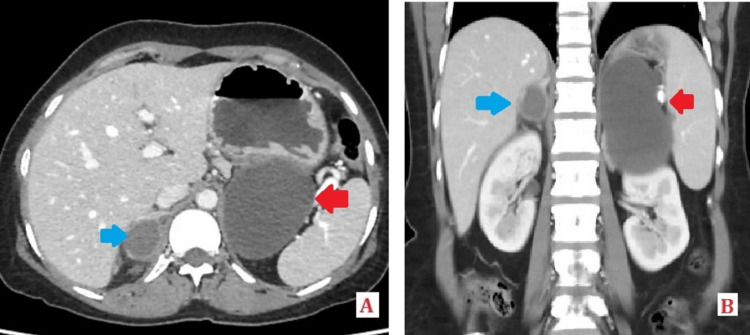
Axial view (A) and coronal view (B) of CECT abdomen with the red arrow showing the non-enhancing cystic lesion over the left adrenal gland region (gastric duplication cyst) and the blue arrow showing the hyperdense peripherally enhancing lesion in the right adrenal gland, suggestive of hemorrhage within the cyst. CECT:  Contrast-enhanced computed tomography
